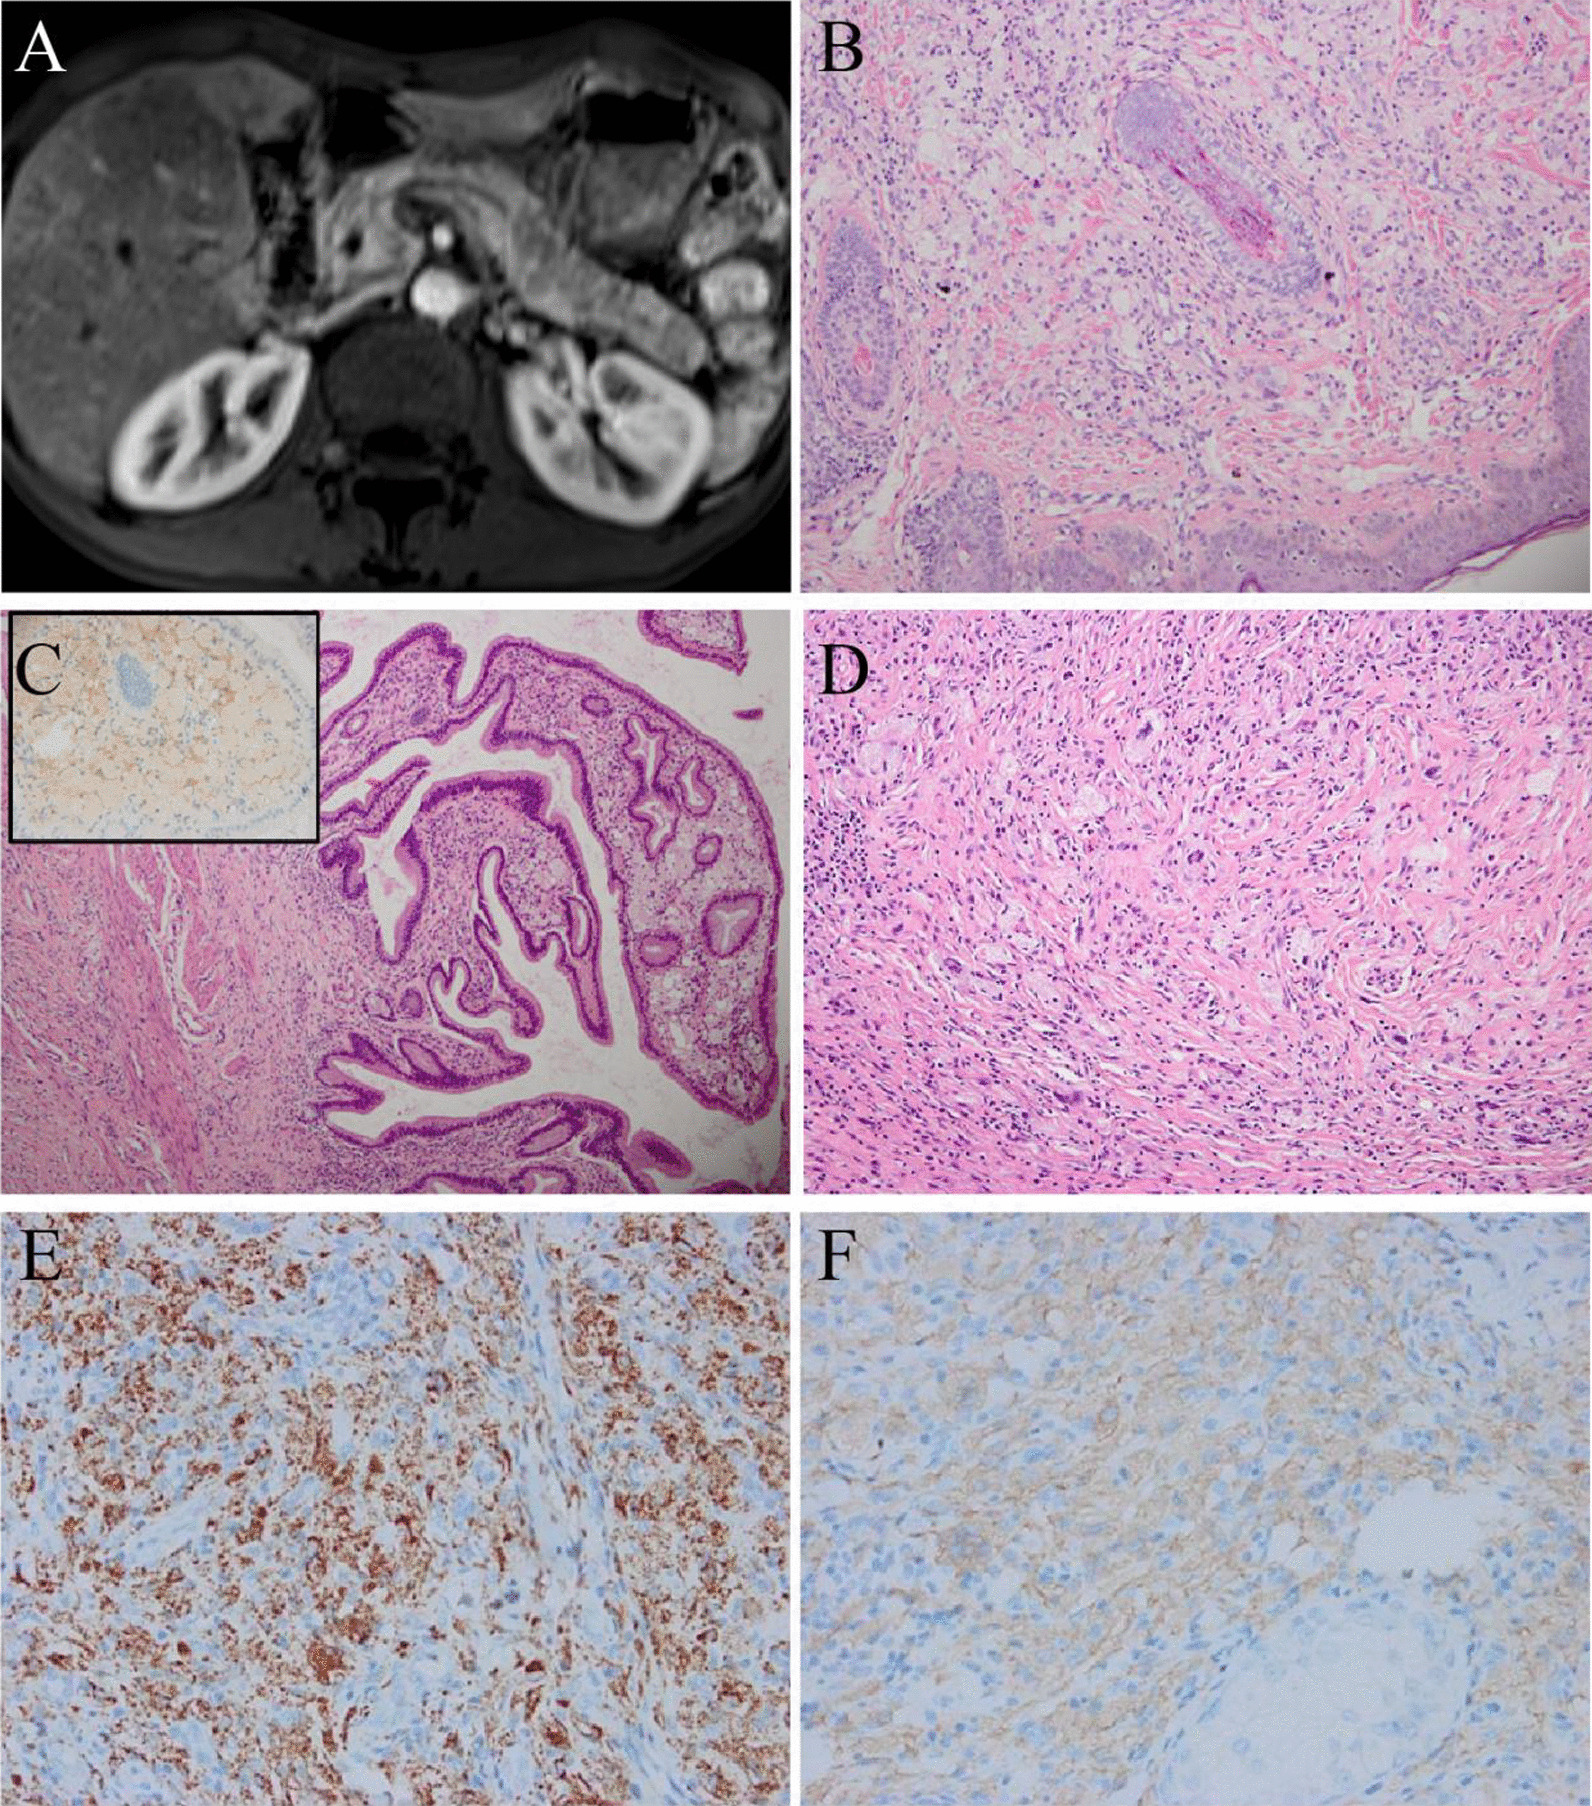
Radiological and pathological features of case 3. A MRI showed multiple patchy and/or nodular abnormal signal shadows in the liver. B The skin biopsy showed dermally infiltrates of histiocytes and Touton cells. C There were numerous foam cells with ALK-positive (inlet) in the mucosal layer of the gallbladder and fibrous tissue hyperplasia in the muscular layer. D The liver lesions showed prominent interstitial sclerosis, accompanied by foamy cells and Touton giant cells. E The histiocytes were positive for CD68 (Skin). F The histiocytes were positive for ALK1 (Skin). (Original magnification × 40 [C]; × 100 [B, D]; × 200 [E, F])
